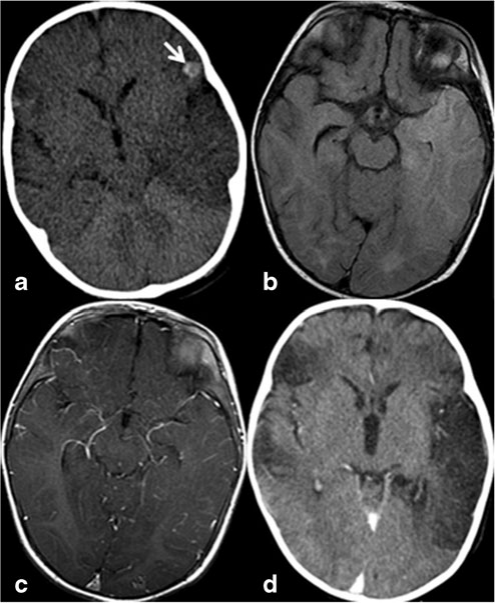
A 7-month-old infant with herpes encephalitis. At presentation (a), asymmetric hypodensity is noted in the left temporal lobe on noncontrast CT and subtle hypodensity in the right temporal lobe. Hemorrhagic focus is noted on the left frontal operculum (*arrow*). MRI at presentation shows bilateral temporal lobe FLAIR hyperintensity left greater than right (b) and leptomeningeal enhancement (c). Delayed contrast-enhanced CT (d) shows development of encephalomalacic changes in the temporal lobes bilaterally, left greater than right and in the right frontal lobe
